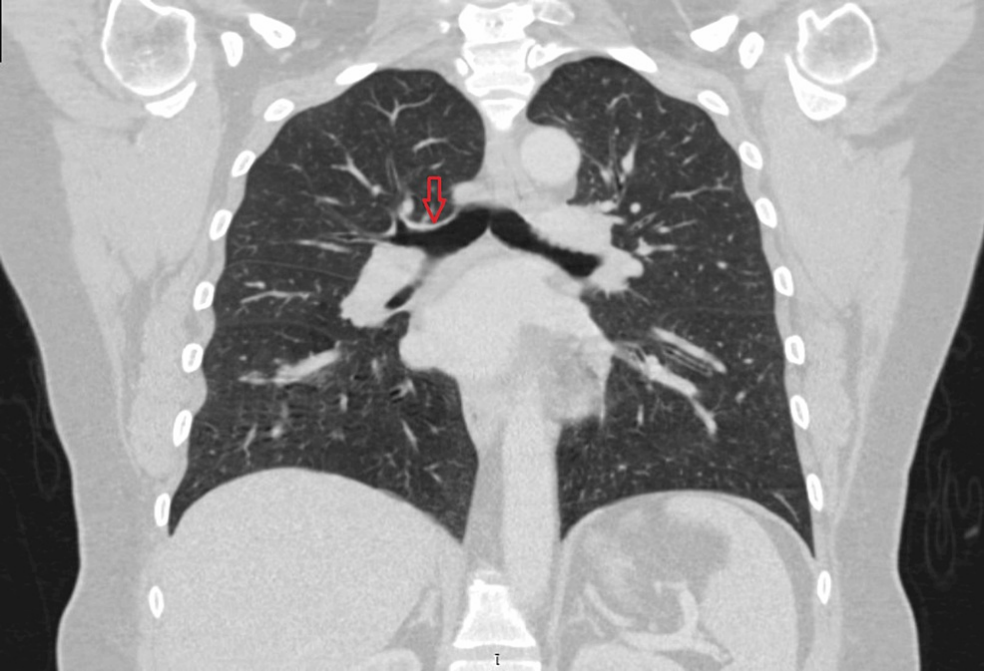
Postprocedural CT scan Arrow pointing towards the previous tumor location.
